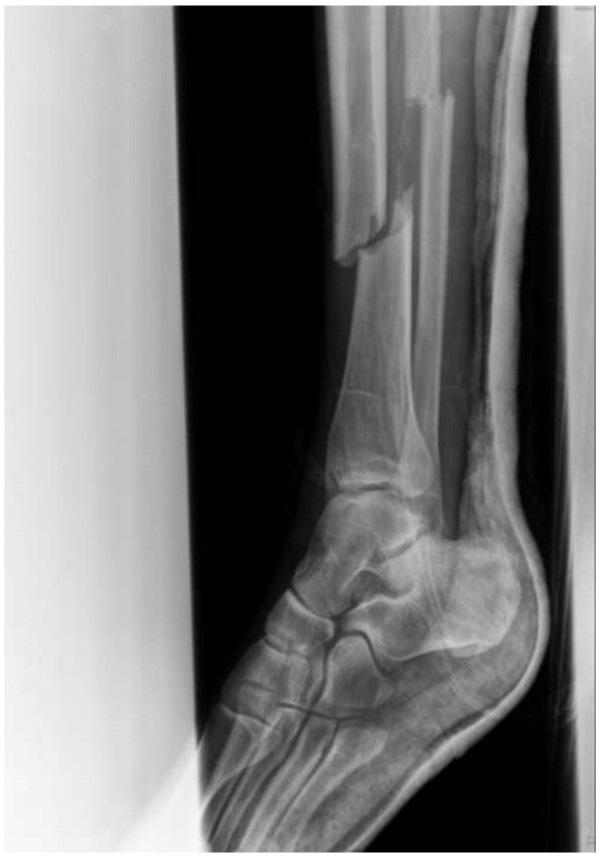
Radiograph of the left tibia at presentation in the emergency room.
